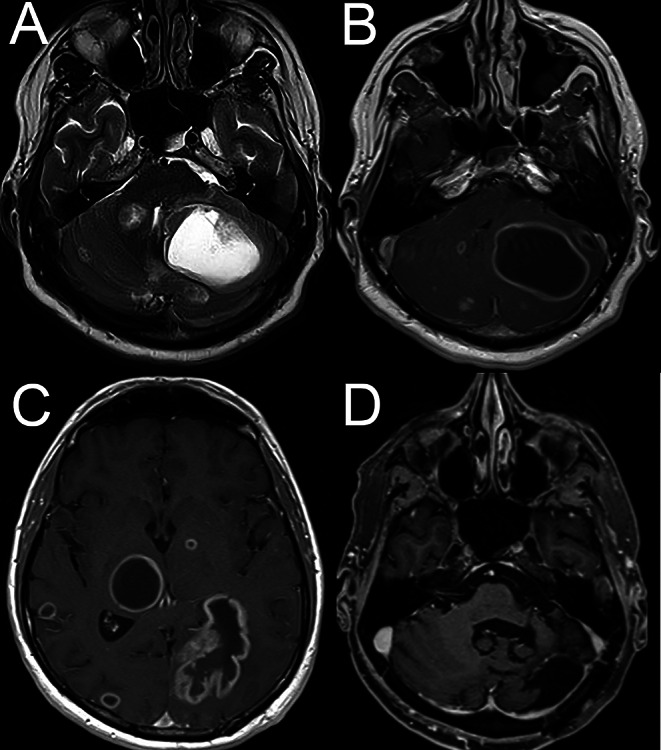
On magnetic resonance imaging (MRI) axial T2-weighted (panel A) and axial T1 post contrast (panel B) images demonstrate multiple cystic cerebellar space-occupying lesions compressing the fourth ventricle. Additionally, multiple supratentorial cystic mass lesions were depicted on MRI (panel C, axial T1 post contrast sequence). At follow-up after 9 months (panel D) a status post left-sided sub-occipital craniotomy and resection of the large left-cerebellar cystic mass lesion can be seen. (Source: Department of Radiology, Cantonal Hospital of Baden, Baden, Switzerland)
